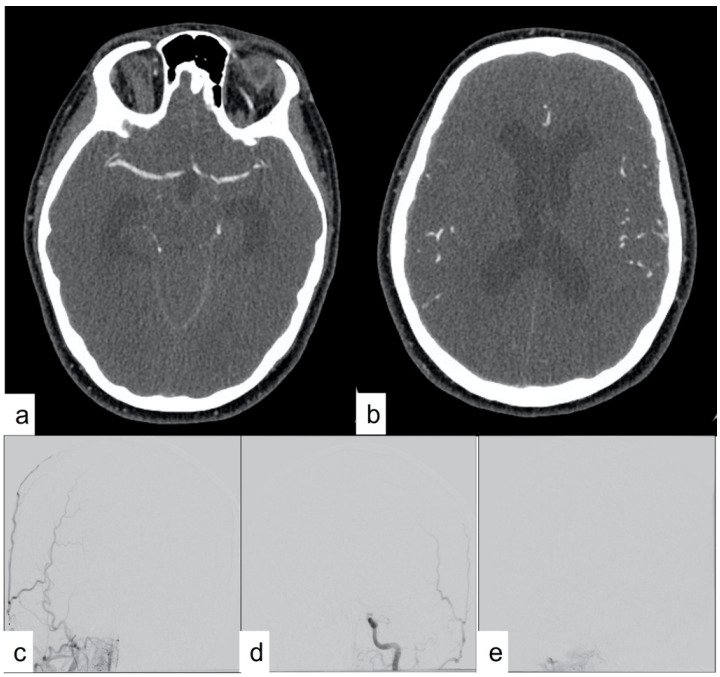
CTA showing opacification of intracranial arteries but no opacification of the deep venous system (a,b). On the same day, a digital subtraction angiogram of the right (c) and left (d) common carotid arteries and dominant right vertebral artery (e) showed no filling of intracranial arteries but continued filling of the extracranial arteries, consistent with findings of DNC. This is based on most of the scoring system criteria, but DNC cannot be confirmed except using the venous score.
